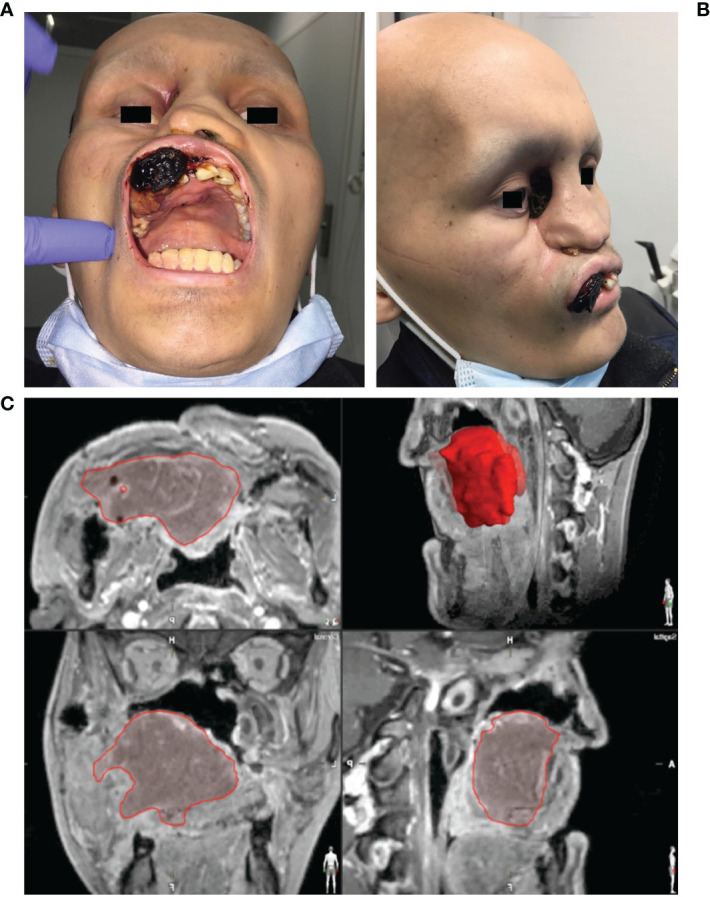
Clinical findings and virtual data in a case of Ewing’s sarcoma of the midface. (A) Frontal/intraoral and (B) side view of a 19-year-old patient with an Ewing’s sarcoma of the midface presenting with an ulcerating neoplasm in the area of the hard palate, the maxillary vestibule and the right medial nasal slope which has lead to a sinking of the right eyeball. (C) Segmented tumor extent (red) in the multi-planar view of the MRI images.
